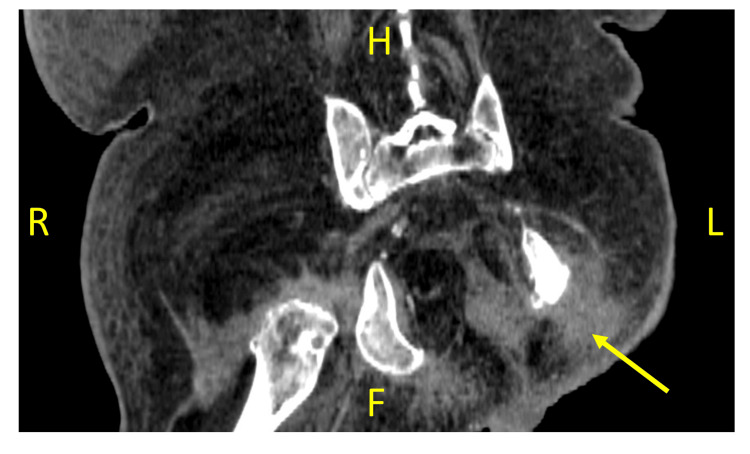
Snapshot from a coronal non-contrast CT scan of the pelvis taken on day 37 of admission. Arrow indicates the site of the previous fluid collection in the left buttock adjacent to the left ischium, which was drained on day 22 of admission H: head, F: foot, R: right, L: left
